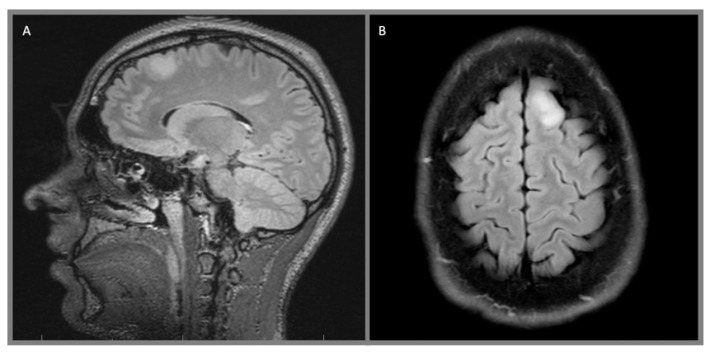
Left frontal diffuse astrocytoma, WHO 2: (A) sagittal T2 Fluid-attenuated inversion recovery (FLAIR) and (B) axial T2 FLAIR sequences demonstrate a diffusely infiltrating, hyperintense lesion in the left superior frontal gyrus.
